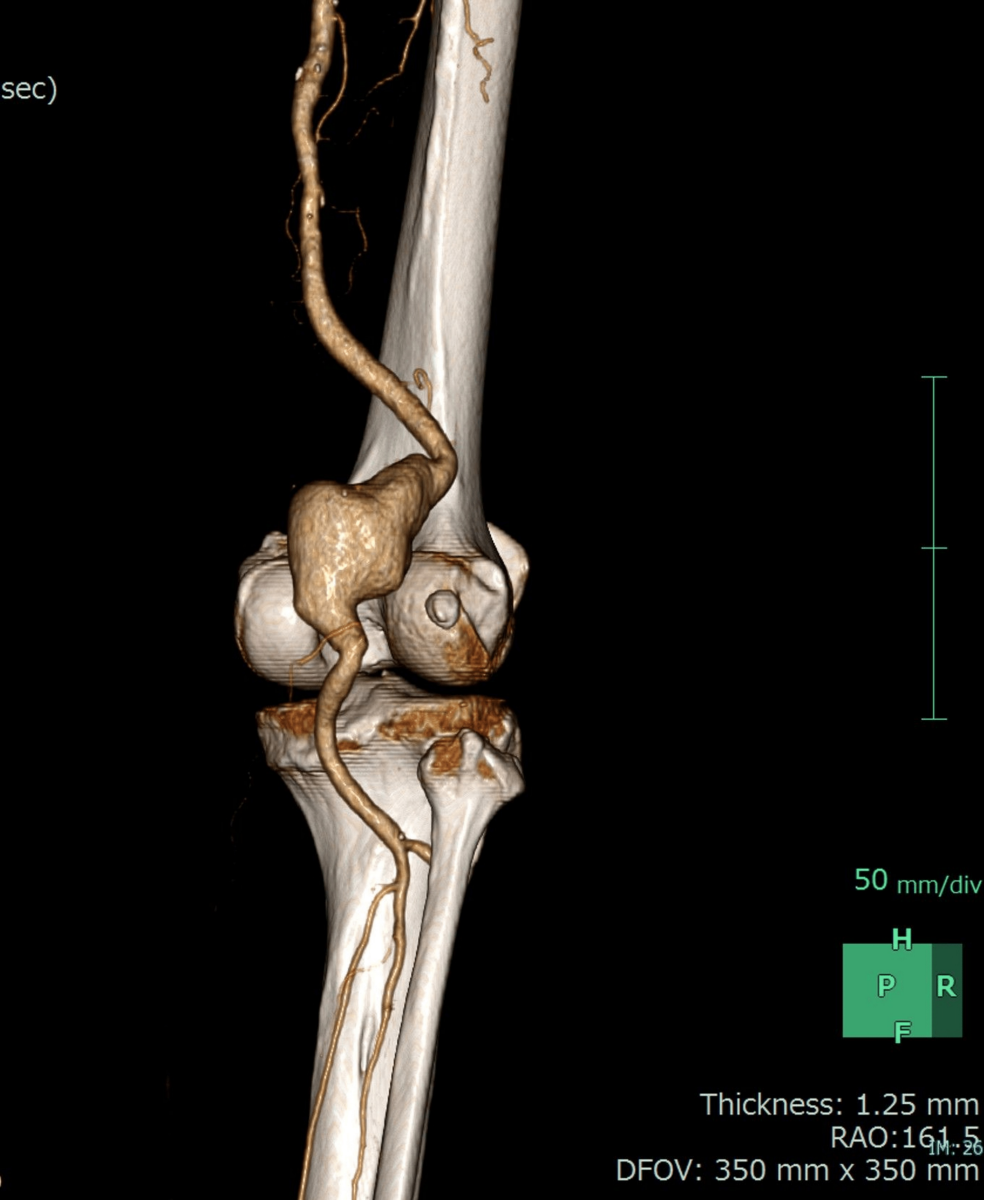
Three-dimensional computed tomography image of the right popliteal artery aneurysm.
